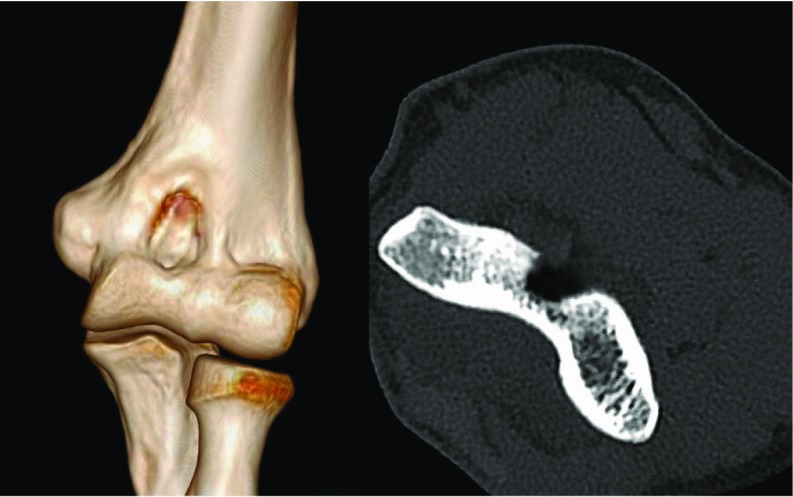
Post-operative computed tomography images showing base after removal of the lesion.
